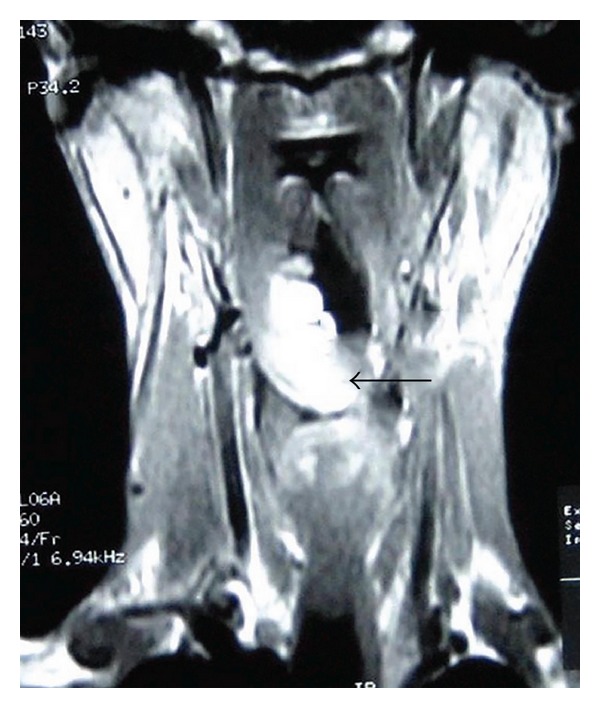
A T2-weighted MRI coronal image showing the hyperintense lesion.
